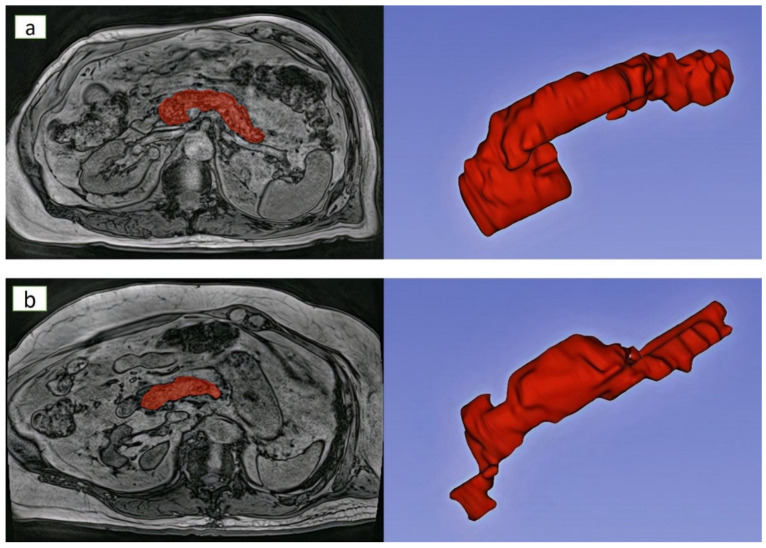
Exemplar segmentation and 3D reconstruction of the pancreas MR images in individuals with (a) healthy pancreas and (b) chronic pancreatitis. *Footnotes:* The healthy individual was a 77-year-old man with a BMI of 27. The individual with chronic pancreatitis was a 77-year-old man with a BMI of 26.
